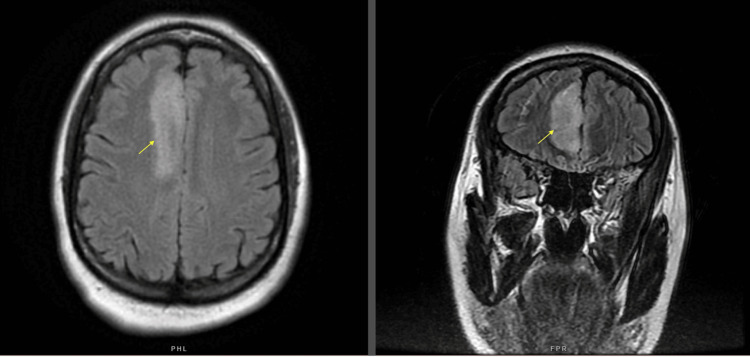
(A) MRI of the brain without IV contrast, axial view, T2 FLAIR PROPELLER, and (B) coronal view, T2 FLAIR, showing a moderate-sized area of restricted diffusion in the right parasagittal frontal lobe, compatible with an acute or subacute infarct located in the right anterior cerebral artery distribution. There is associated edema, swelling, and local mass effect, with sulcal effacement and slight right-to-left deviation of the frontal lobe by approximately 2 mm. No other areas of acute infarction are seen. Yellow arrows indicate the area of restricted diffusion corresponding to the infarct. FLAIR: Fluid-attenuated inversion recovery.
